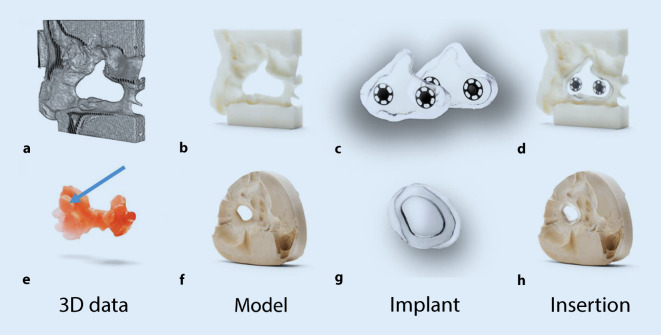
Manufacturing process for nasal septal buttons. a–d Computed tomography scans, e–h silicone impression
